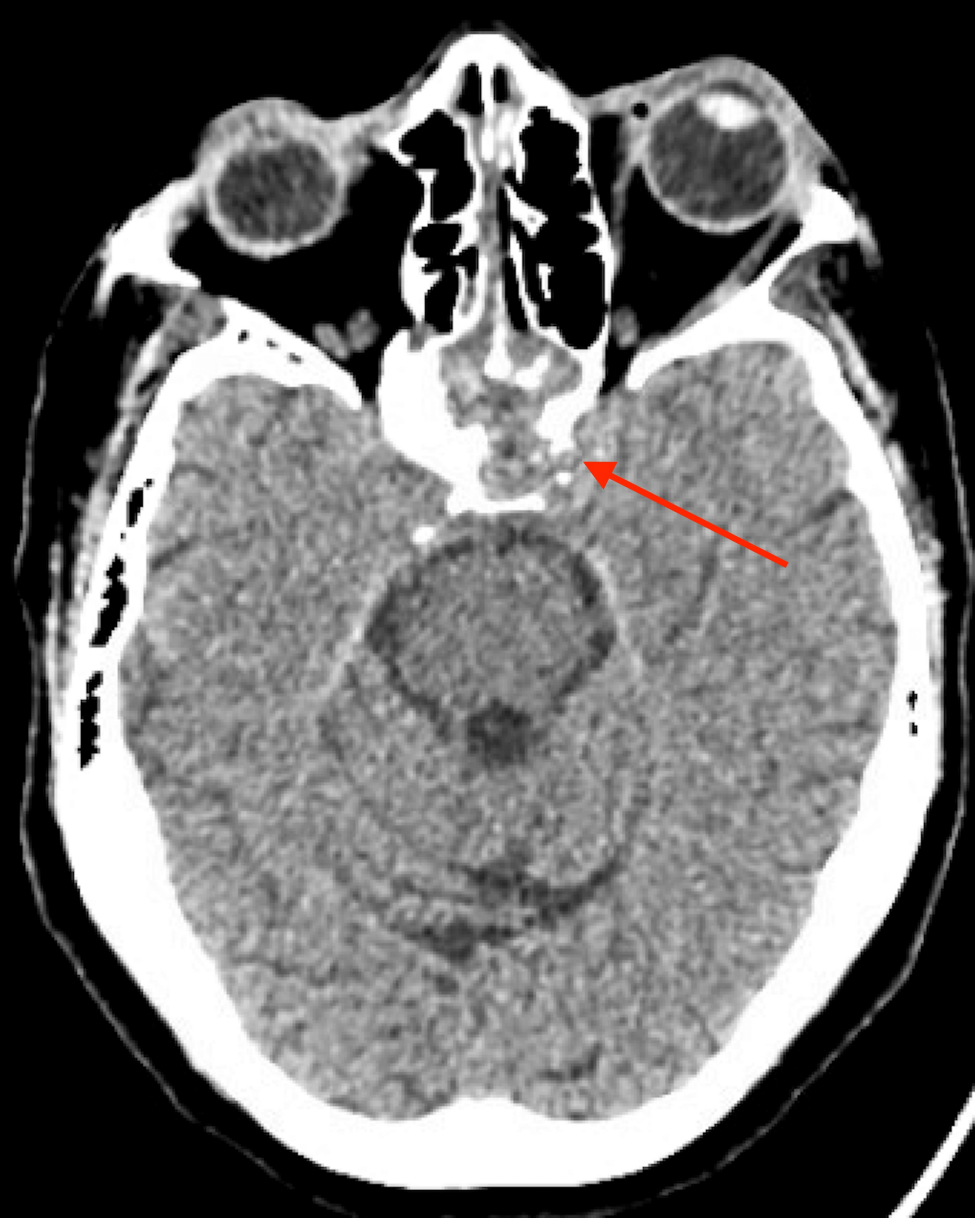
Axial CT image showing complete opacification of the bilateral sphenoid sinuses CT head without contrast demonstrates opacification of the sphenoid sinuses with associated osteogenesis and dehiscence of the posterior wall of the sphenoid sinus along the anterior wall of the sella.
